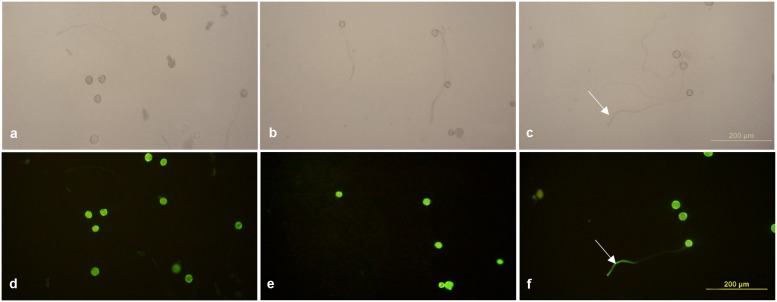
Expression of *eGfp* in pollen tubes germinated from transformed pollen grains. (a,d) Fresh pollen grains suspended in 15% sterilized sucrose solution; (b,e) germinated pollen grains incubated with recombinant plasmid containing *eGfp* gene without ultrasonication treatment applied; (c,f) germinated pollen grains incubated with recombinant plasmid containing *eGfp* with ultrasonication treatment applied. (a–c) Photographed under bright field with white light illumination; (d–f) photographed under blue light illumination. *EGfp* expression is visible in a pollen tube (white arrows shown in c,f) of the ultrasonication treated pollen grains, whereas no *eGfp* expression was observed in the pollen tubes of untransformed pollen grains, although pollen tubes are clearly visible under while light illumination. Pollen suspension from all treatment groups were incubated at 25°C.
